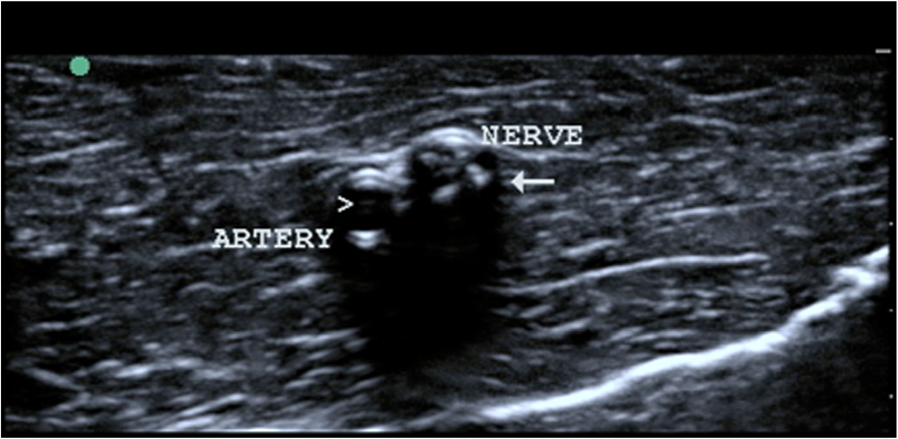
Ultrasonographic image of the nerve and artery.
